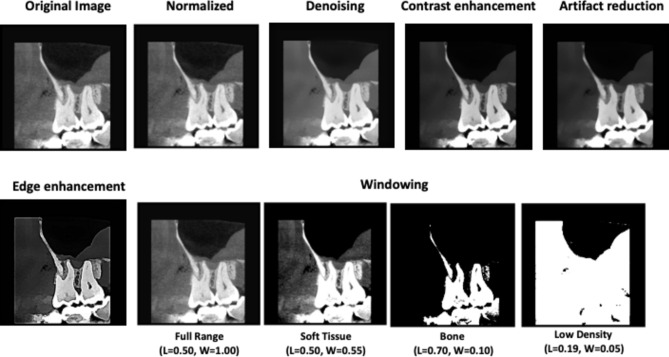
Digital image pre-processing stages of grey-scale radiographs.
